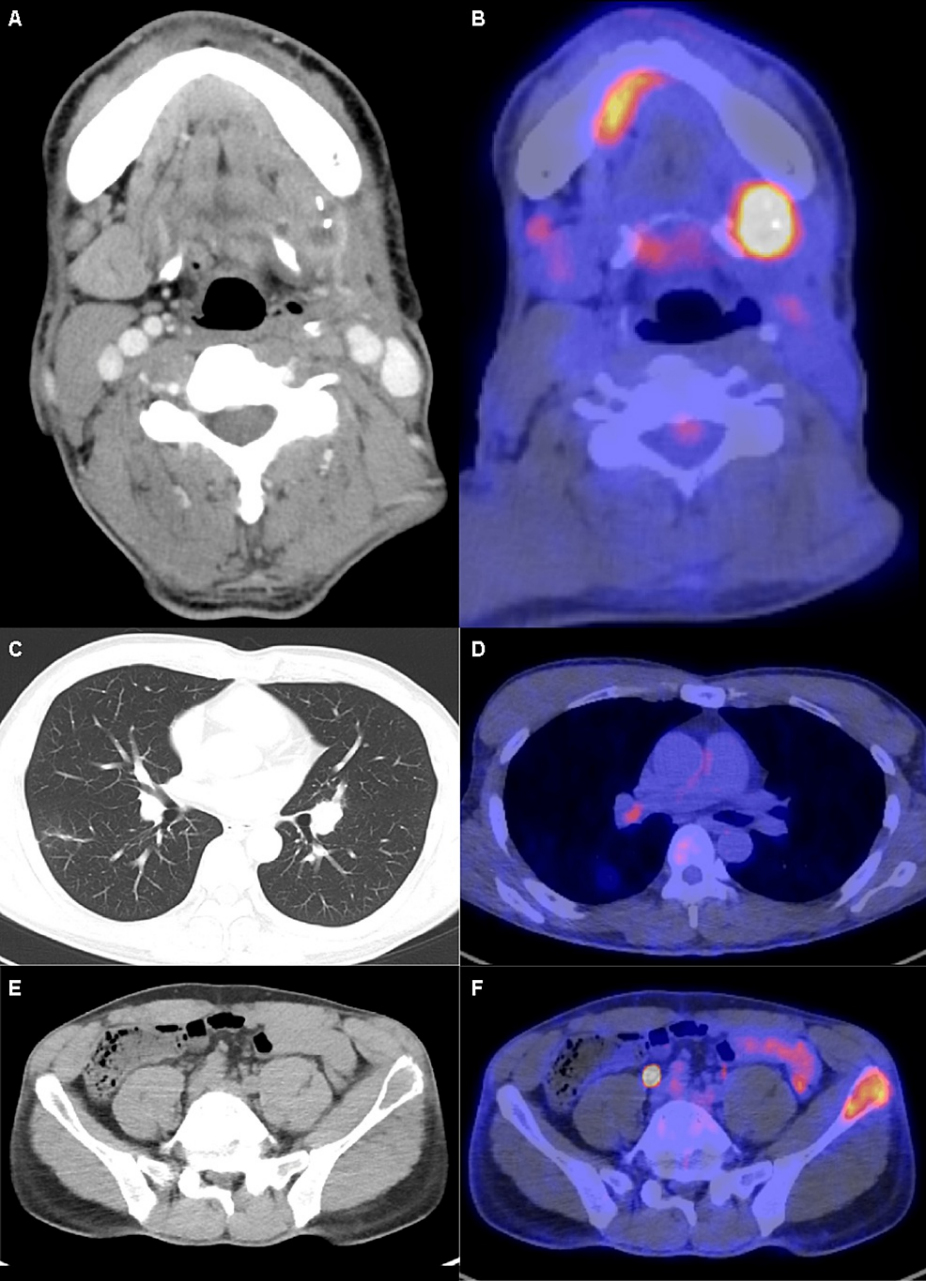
Radiological findings of the tumor in Case 1 before nivolumab administration. Findings before nivolumab administration. Computed tomography (CT; left) revealed a swelling in the left submandibular region (A, B), pale ring-shaped nodular shadows in both the lung fields (C, D), and osteolytic changes in the left iliac bone (E). Fluorodeoxyglucose-positron emission tomography (FDG-PET; right) revealed an abnormal accumulation in the left iliac bone (F).
